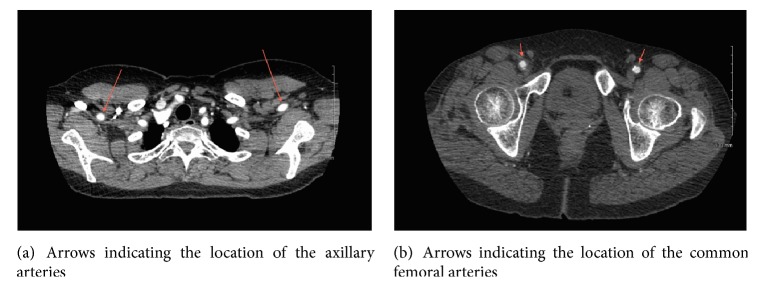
Contrasted enhanced CT scan images.
